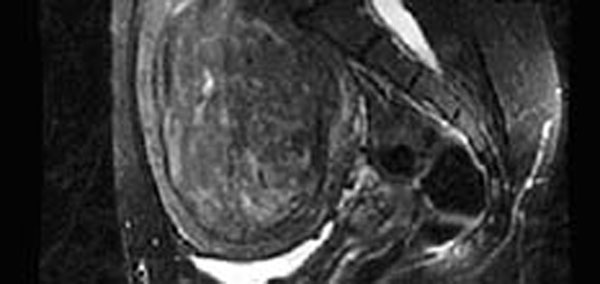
Sagittal T2-weighted image depicting a 471 cc heterogeneously hyperintense fibroid.
